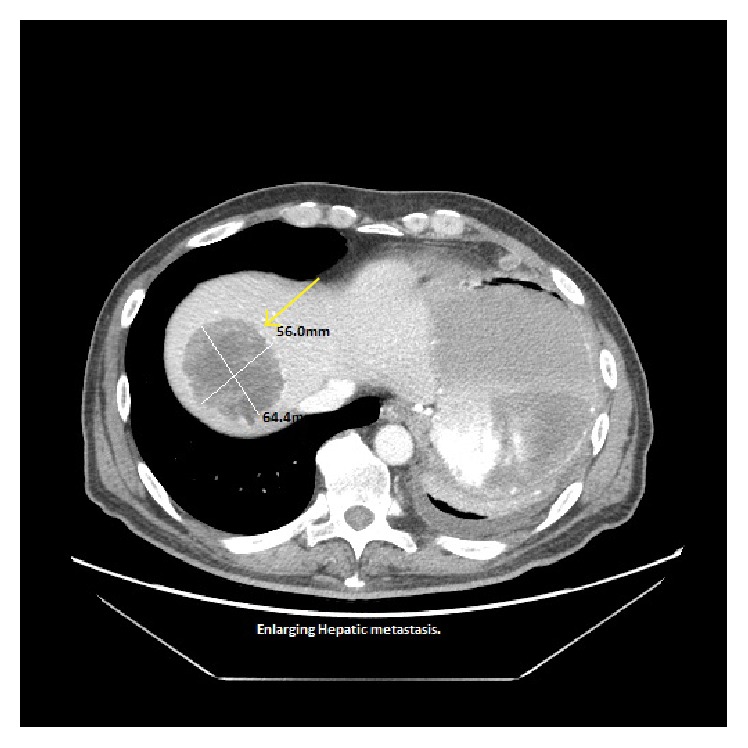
Previous CT (contrast enhanced) showing liver metastases (yellow arrow) smaller at that time and no perihepatic fluid.
